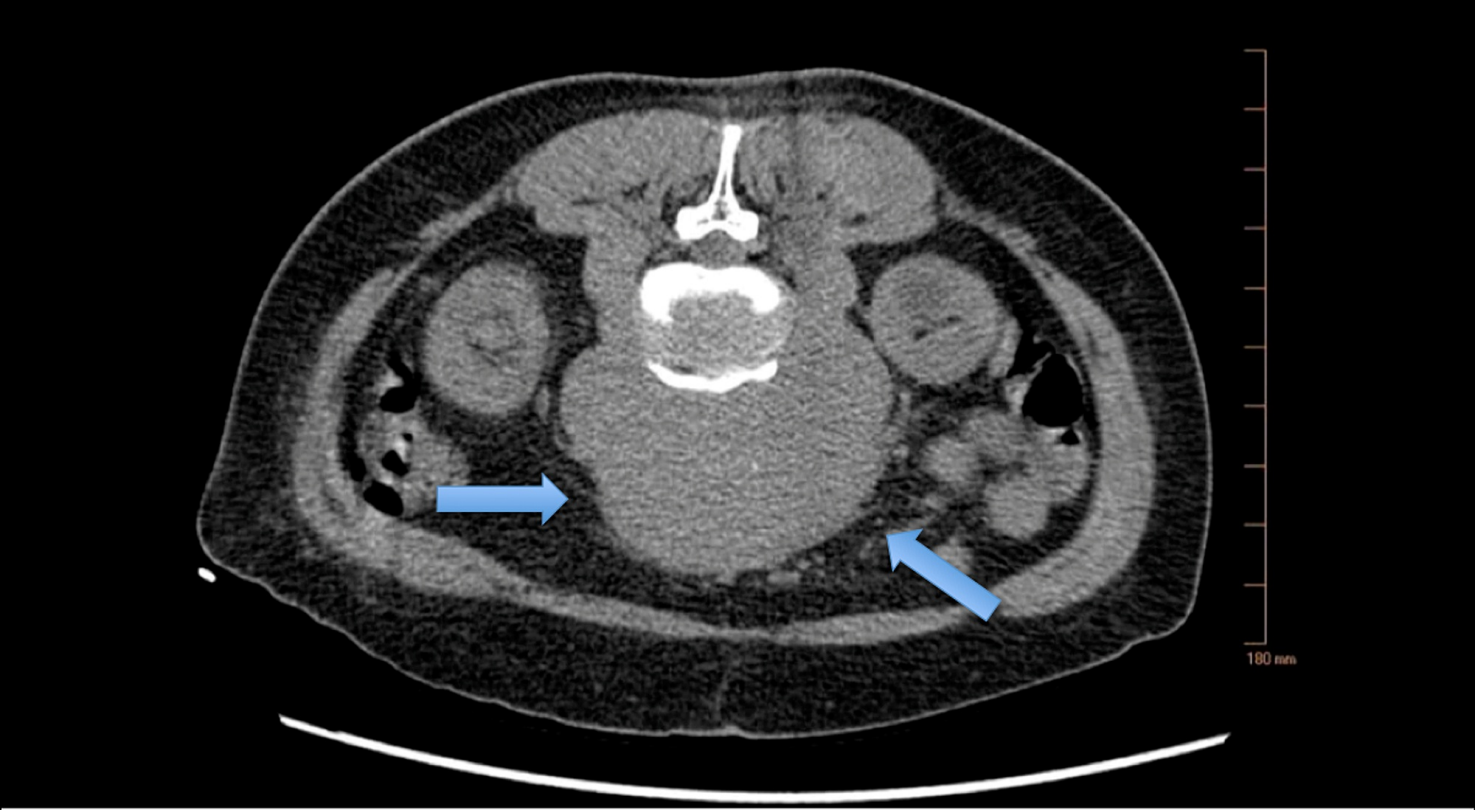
CT scan shows the large retroperitoneal mass.
